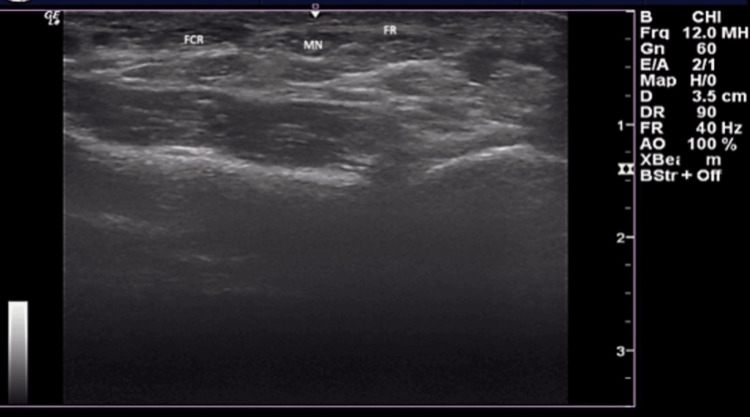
Confirmed Absence by Ultrasound Ultrasound did not detect the palmaris longus (PL) in this image. No presence of a fusiform muscle was noted superficial to the flexor retinaculum (FR). Additionally, relevant anatomical structures, such as the median nerve (MN) and flexor carpi radialis (FCR), are labeled in this figure.
